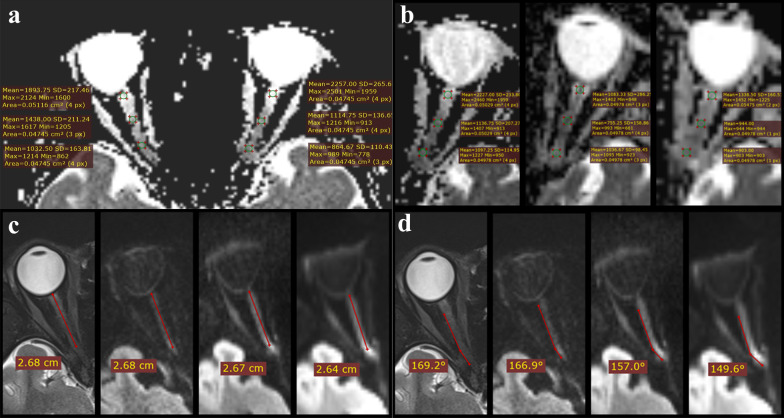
Examples of quantitative optic nerve measurements. a Measurement of ADC values. Three ROIs were measured on each side. b, Measurement of ADC values. Three ROIs were measured on the left side, along with (left to right) images of ADC map of rs-EPI DWI, ADC map of rFOV-EPI DWI and ADC map of ss-EPI DWI, respectively; c the length of the intraorbital segment of the optic nerve is shown, along with (left to right) images of T2w, rs-EPI DWI at b value = 1000 s/mm^2^, rFOV-EPI DWI at b value = 1000 s/mm^2^ and ss-EPI DWI at b value = 1000 s/mm^2^ (respectively); d Maximum angles of the optic nerve are shown, along with (left to right) images of T2w, rs-EPI DWI at b value = 1000 s/mm^2^, rFOV-EPI DWI at b value = 1000 s/mm^2^ and ss-EPI DWI at b value = 1000 s/mm^2^ (respectively). Compared with the T2w images, the DWI images had different degrees of distortion, and the angle of the optic nerve became smaller. ADC, apparent diffusion coefficient; rs-EPI DWI, readout-segmented echo-planar imaging diffusion-weighted imaging; rFOV, reduced field-of-view; ss-EPI, single-shot echo-planar imaging
